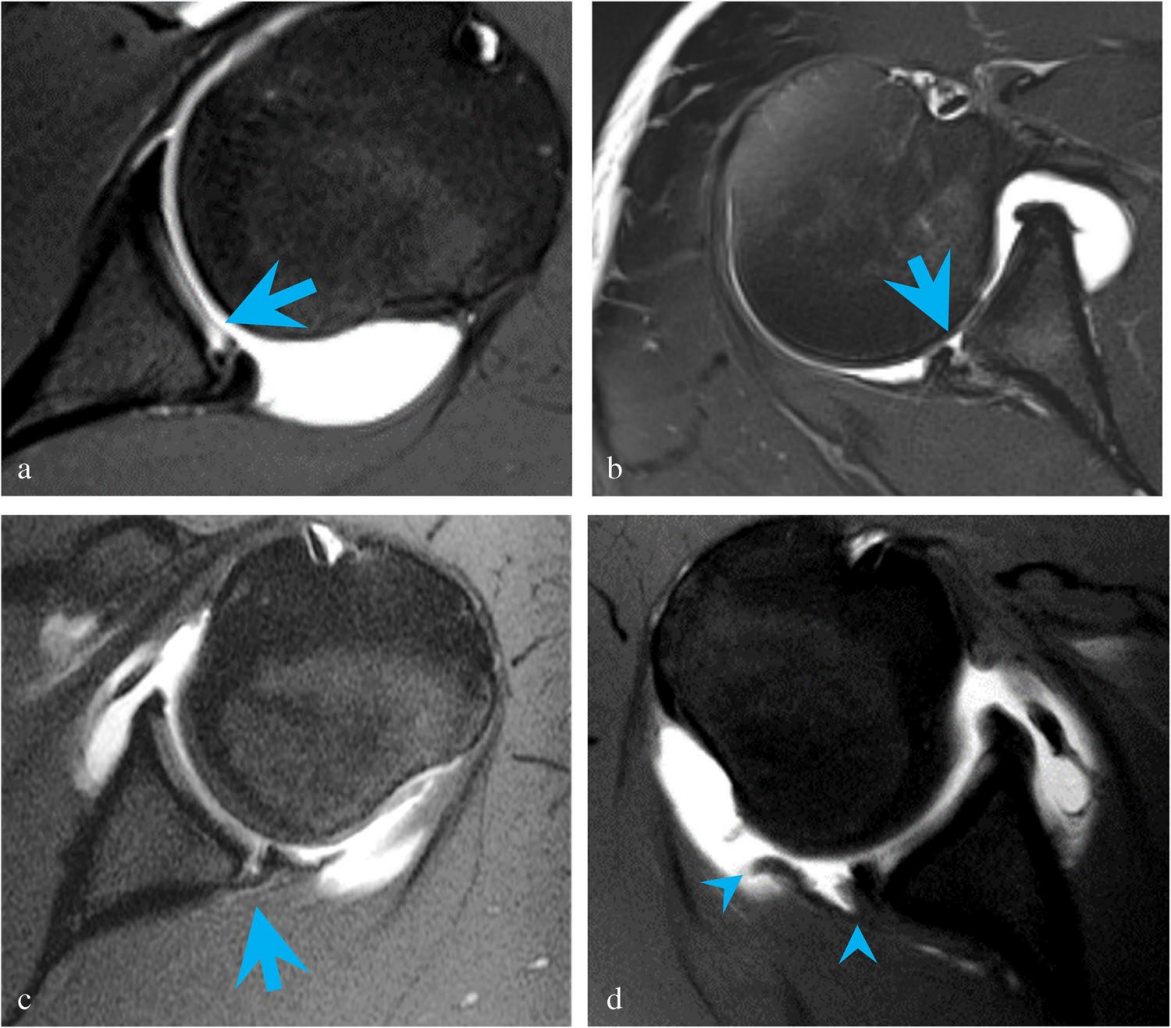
a Axial T1-weighted image with fat suppression demonstrates posterior glenolabral articular disruption (PLAD) with tear of the articular cartilage (blue arrow) in a 17-year-old male pitcher with 6 months of pain and instability following an acute subluxation event during batting. Arthroscopy showed a tear of the posterior labrum extending from 6-o’clock to 9-o’clock position with fraying of the posteroinferior glenoid cartilage. b Axial T2-weighted image with fat suppression shows a reverse Bankart lesion (blue arrow) in a 32-year-old male with pain and instability following a hockey injury. A slightly displaced bony fragment was found at surgery. The posterior labrum was noted to be attached to the bony fragment but torn away from the remaining glenoid. c Axial T1-weighted image with fat suppression shows a posterior labrocapsular periosteal sleeve avulsion (POLPSA) with stripping of the periosteum (blue arrow) in an 18-year-old male with 6 months of pain and recurrent subluxations following an acute dislocation event with self-reduction playing football. Arthroscopy confirmed a posterior labral tear extending from 7 o’clock to 9 o’clock. d Axial T1-weighted image with fat suppression demonstrates a posterior humeral avulsion of the glenohumeral ligament (PHAGL) (avulsed ligament delineated by blue arrowheads) in a 17-year-old male with 3 months of pain and recurrent subluxations following an acute posterior dislocation event while checking another player during a hockey game. Arthroscopy confirmed a tear from the 6 o’clock to 10 o’clock position. The posterior capsule and posterior band of the inferior glenohumeral ligament were found to be avulsed from the insertion on the humeral head
